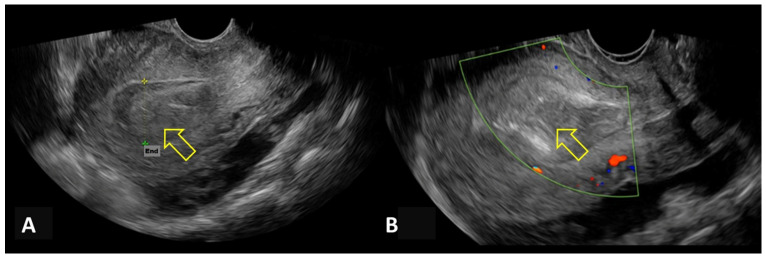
Initial transvaginal ultrasound examination in a patient with post-abortion bleeding. (A) Gray-scale ultrasound showing mixed hypo- and hyperechogenic material within the endometrial cavity, compatible with clot or necrotic tissue (indicated by a yellow arrow); End: endometrial thickness. (B) Absence of abnormal Doppler flow on initial evaluation (indicated by a yellow arrow).
